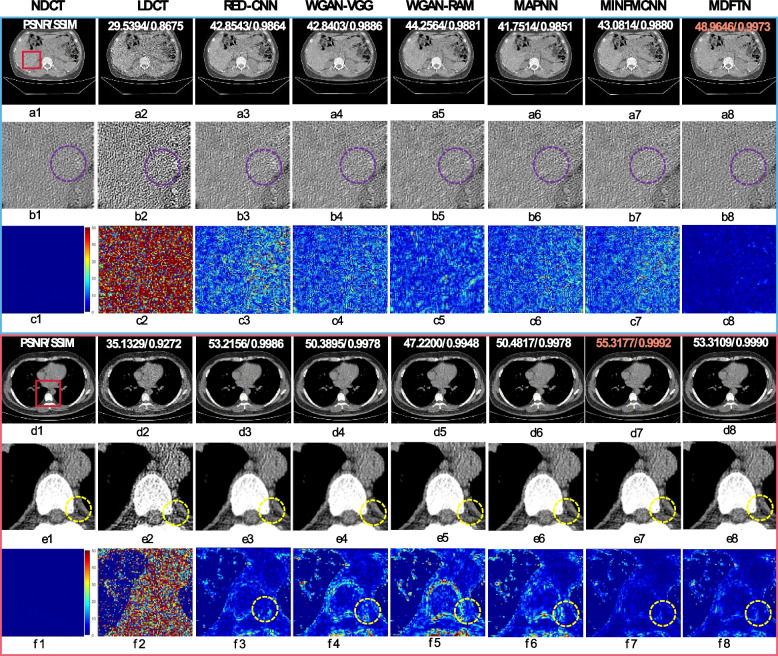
Results and magnified views of data from the synthesized Minfound dataset and RPLHR-CT dataset are provided for comparison. The corresponding ROI for each predicted image is shown below itself. The difference images between the predicted results of different methods and NDCT are labeled as (c1-c8) and (f1-f8), respectively. The orange number represents the highest quantitative index. The purple circles denote flat regions for noise suppression analysis, while the yellow circles highlight detailed structures for visual comparison. The display window range is set at [-160, 240] HU
